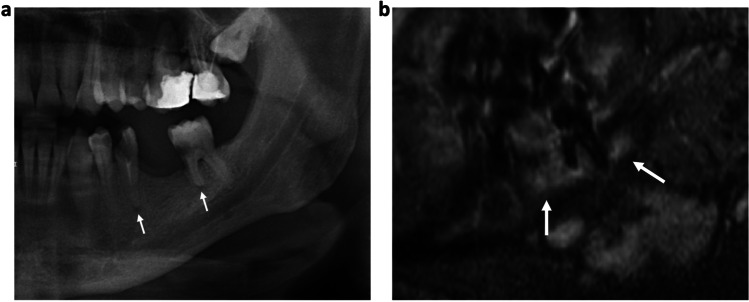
a OPT of a 66-year-old patient with a carious lesion and periodontal disease of the tooth 36. Note the small periapical lesion on the conventional OPT (white arrow). On the sagittal reconstruction of the 3D STIR sequence (b), an extensive alveolar bone marrow edema (arrows) is detected which might indicate a markedly larger extent of the inflammation. Compared to MRI, the extent of inflammatory reaction may be underestimated by conventional OPT
